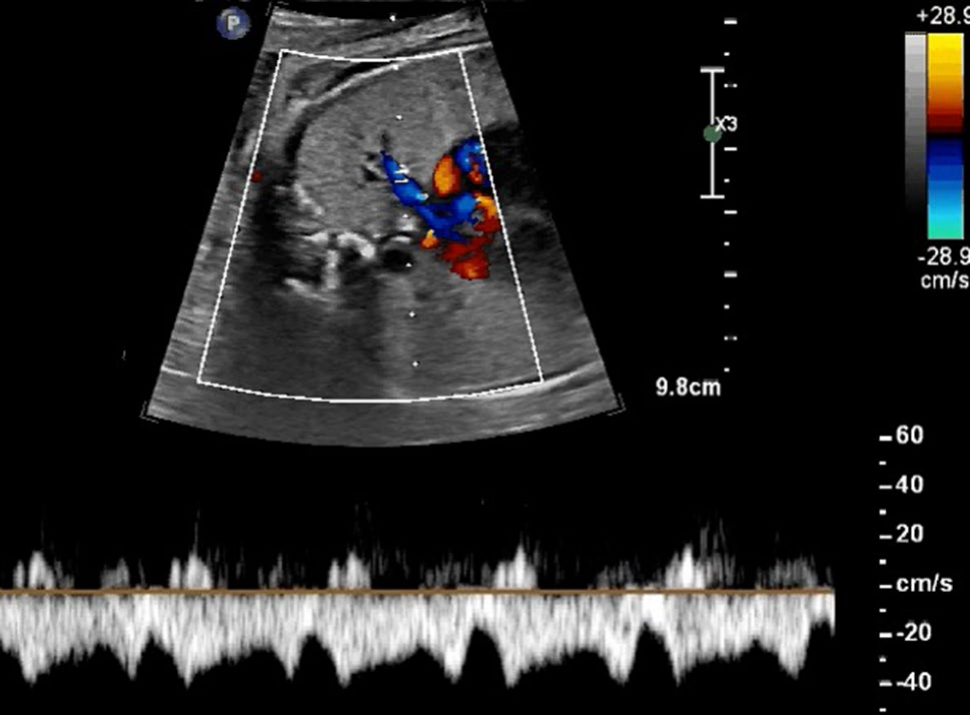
Maximum Doppler flow velocity in the pulmonary vein
